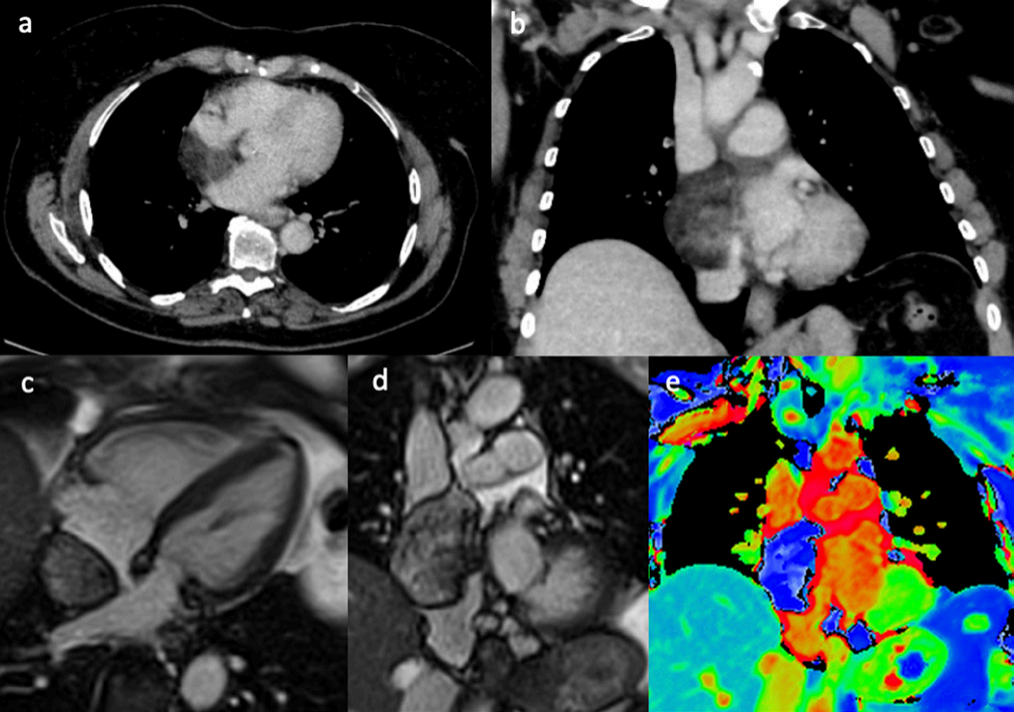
(a & b) Contrast enhanced CT showed an extra atrial but intra
